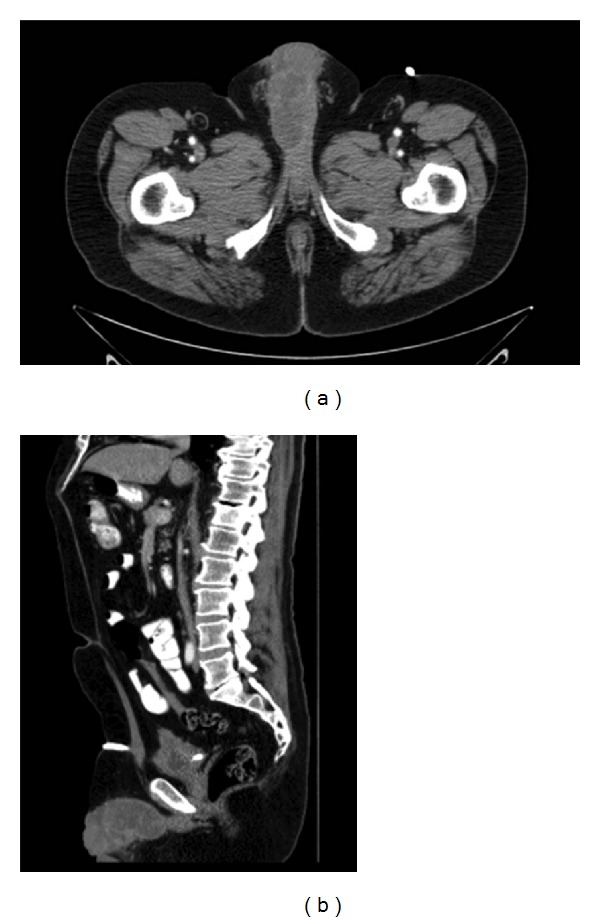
CT scan of abdomen and Pelvis with contrast showing the primary tumor. (a) Transverse view; (b) sagittal view.
